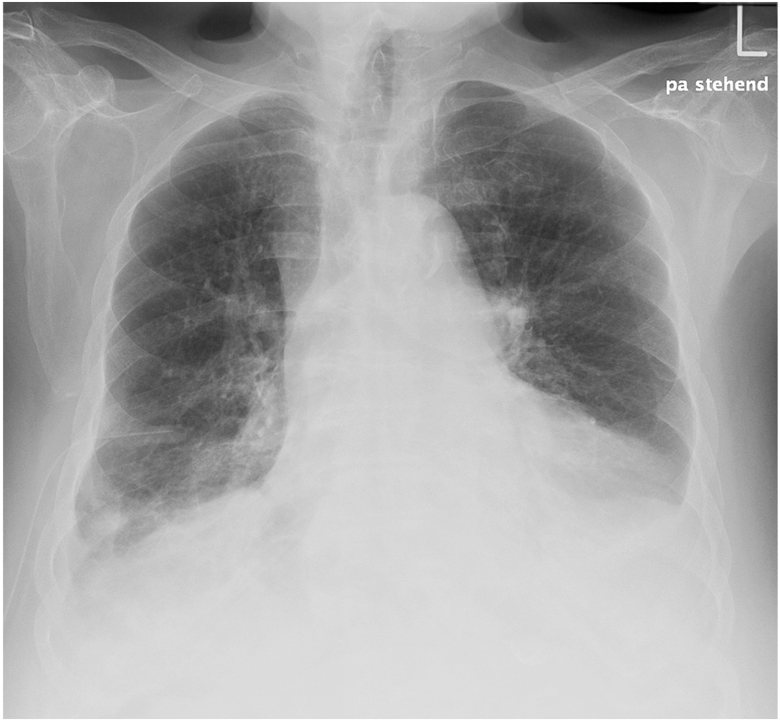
Postoperative radiograph after robot-assisted thoracic surgery diaphragmatic repair. ‘pa stehend’ refers to a posterior–anterior chest X-ray taken in the standing position.
